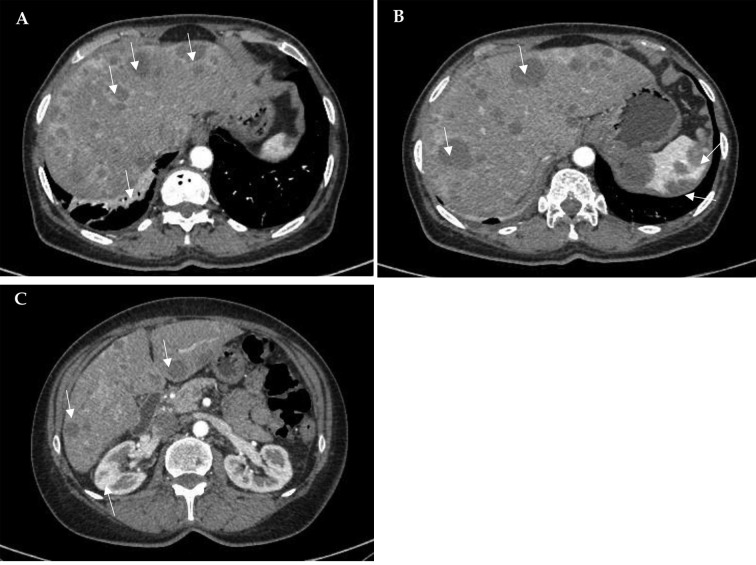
Computed tomography images.* Multiple hypodense solid diffusely distributed masses (*arrows*) in the liver (A–C), spleen (B) and kidneys (C); disc atelectasis in the right lung (*arrow*) (A).
